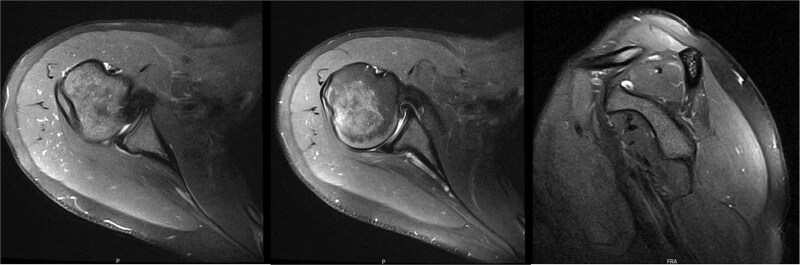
MRI at final follow-up demonstrated mild supraspinatus tendinosis, and infraspinatus atrophy with continued significant fatty infiltration, unchanged from prior imaging.
